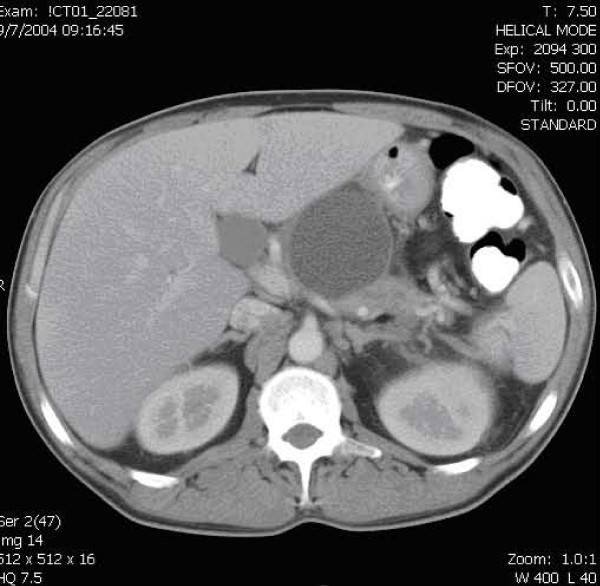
CT of the abdomen showing a large 5.6 × 5.8 cm fluid collection contiguous with the body of the pancreas.
